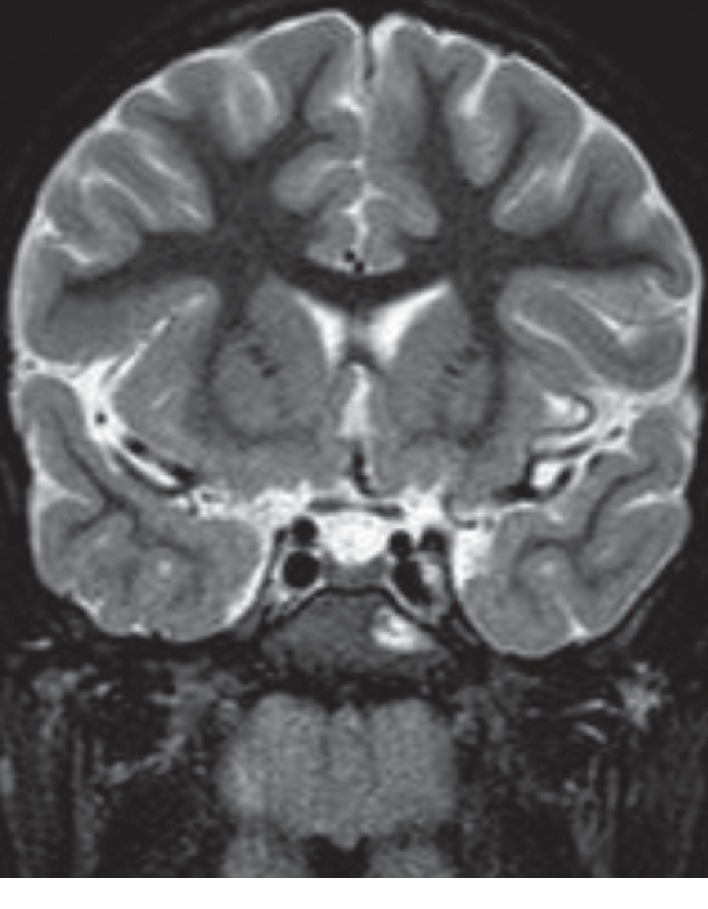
Coronal T2-weighted image reveals an expanded and heterogeneous cavernous sinus.
